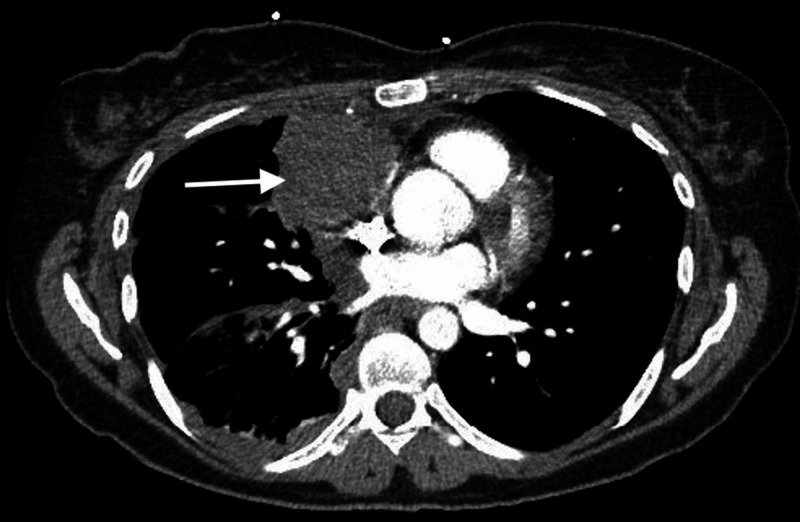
CT chest with arrow indicating right anterior mediastinal mass
